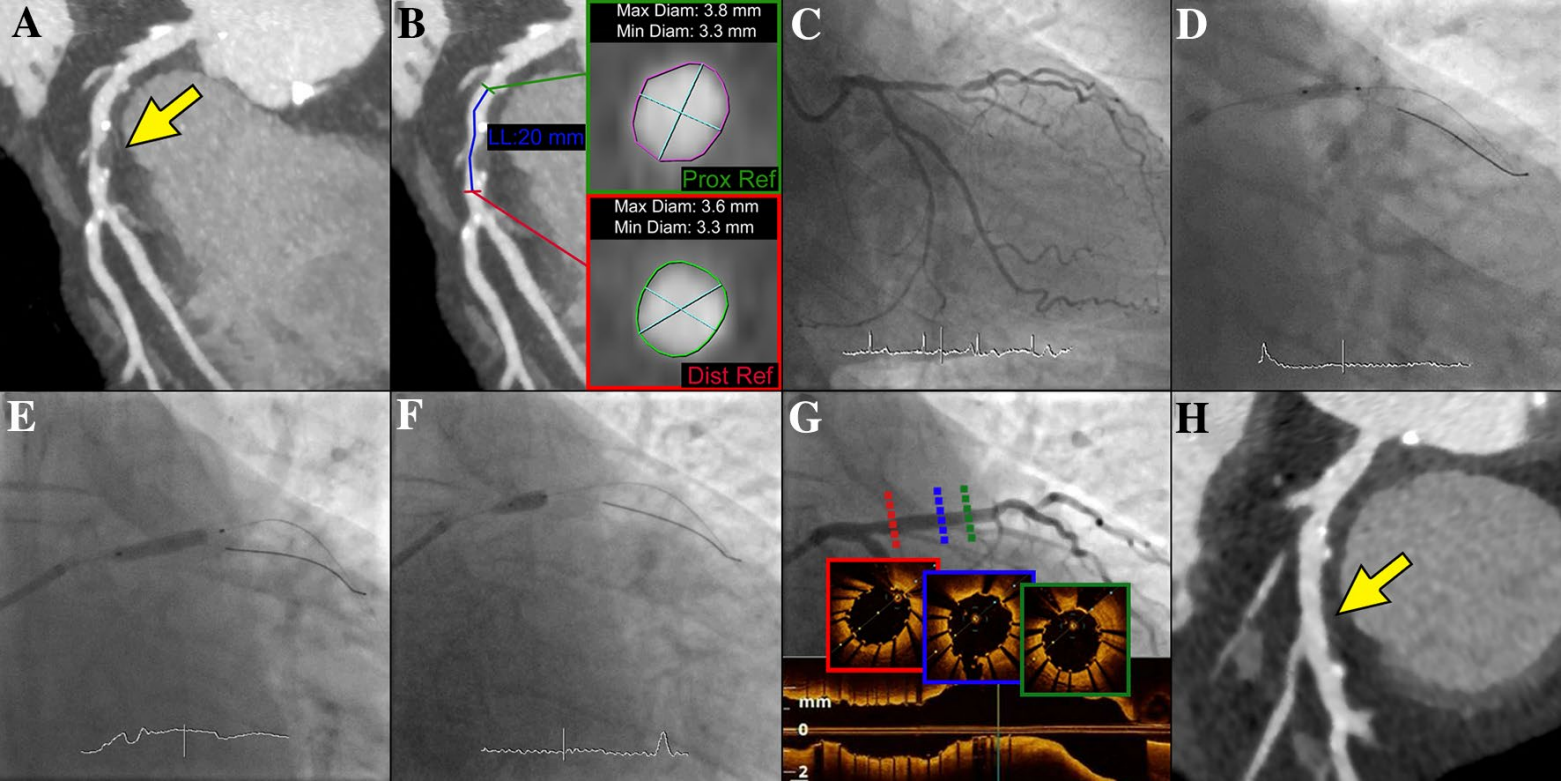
a Coronary computed tomography angiography (CTA) disclosing severe stenosis (arrow) in the proximal-to-mid left anterior descending artery (LAD) (Online Videos 1 and 2). b CTA-assisted planning of percutaneous coronary intervention (based on computed tomographic data the planned stent length was 20 mm with proximal and distal mean stent diameters of 3.5 mm). c Coronary angiography confirming severe stenosis in the proximal-to-mid LAD (Online Video 3). d Balloon predilation of the LAD using non-compliant high-pressure balloon (Pantera LEO 3.0 × 12 mm). e Magnesium-bioresorbable scaffold (Magmaris 3.5 × 20 mm) implantation in the proximal-to-mid LAD with guidewire protection of the diagonal branch. f Proximal optimization technique using non-compliant balloon (NC Quantum 3.5 × 8 mm) in the proximal portion of the scaffold. g Final coronary angiography and optical coherence tomography showing optimal angiographic result with complete apposition and good expansion of the scaffold (Online Videos 4 and 5). h Coronary CTA demonstrating mild atherosclerosis without discernible scaffold struts (arrow) in the proximal-to-mid LAD at 7-month follow-up
